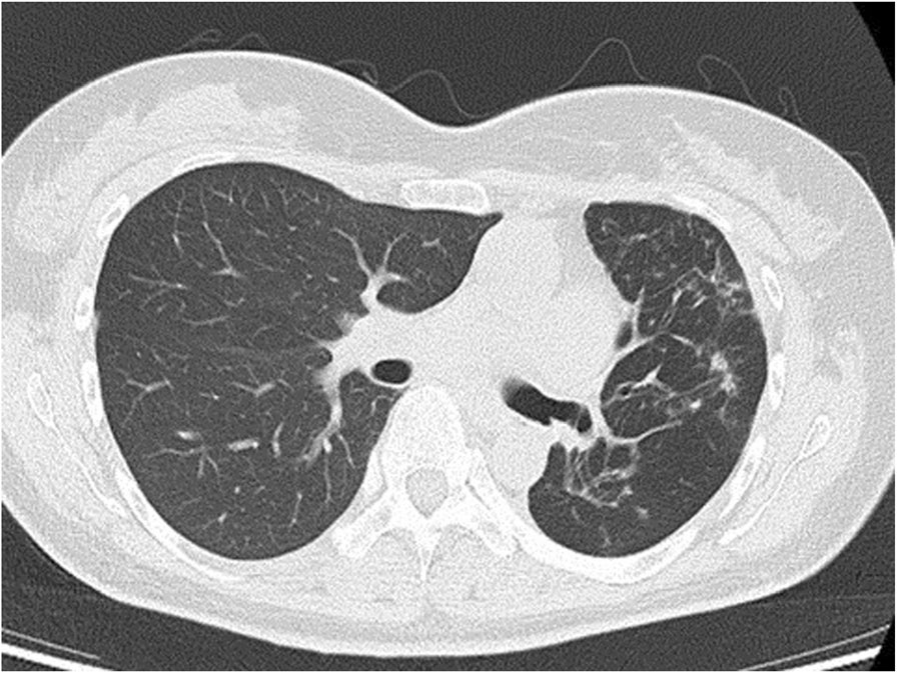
Axial chest CT reveals that the left upper bronchus obstruction was released 4 months after the procedure
